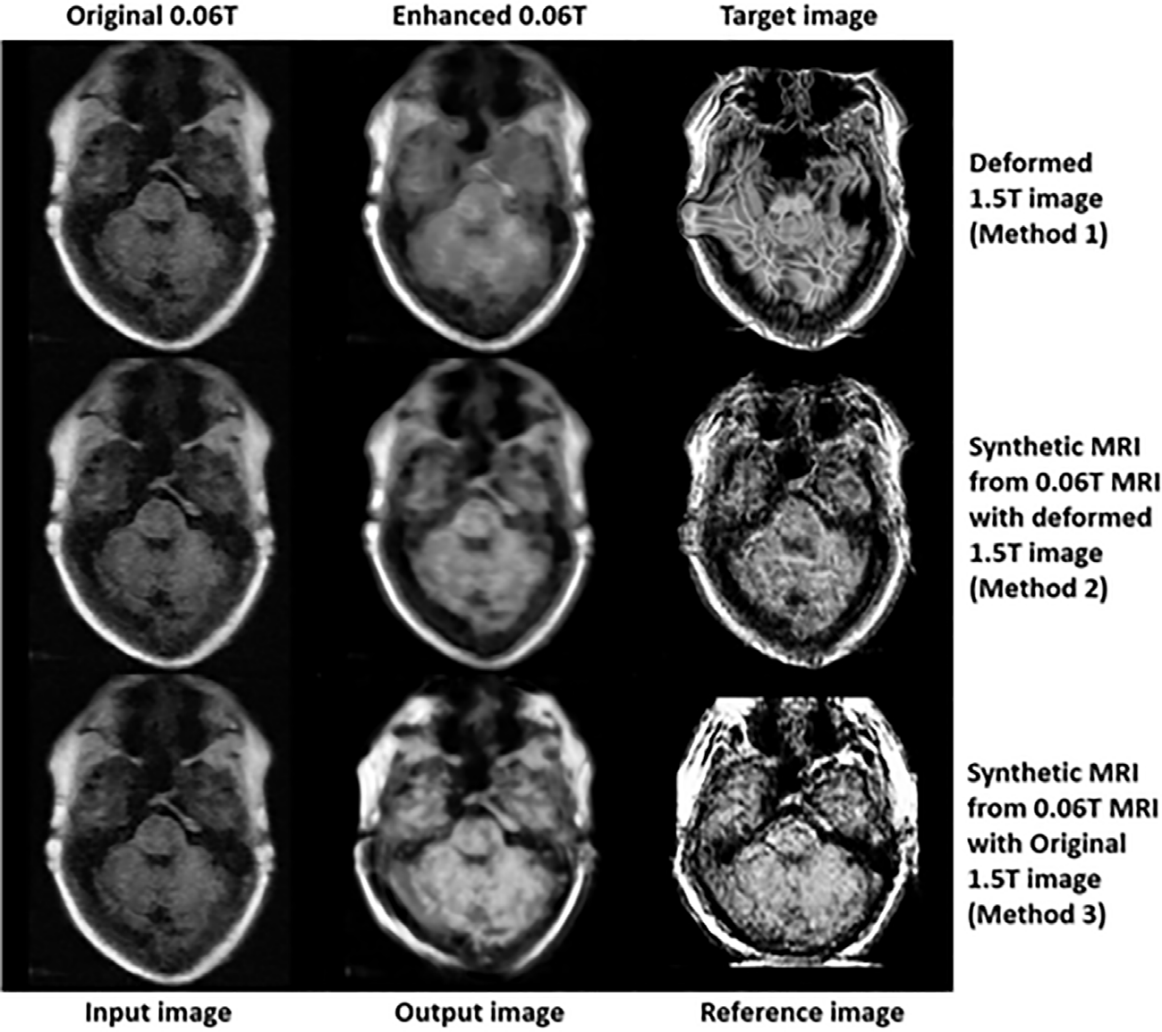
Results of enhanced MRI of slice no. 8 in step 3 (conventional-GAN model).
